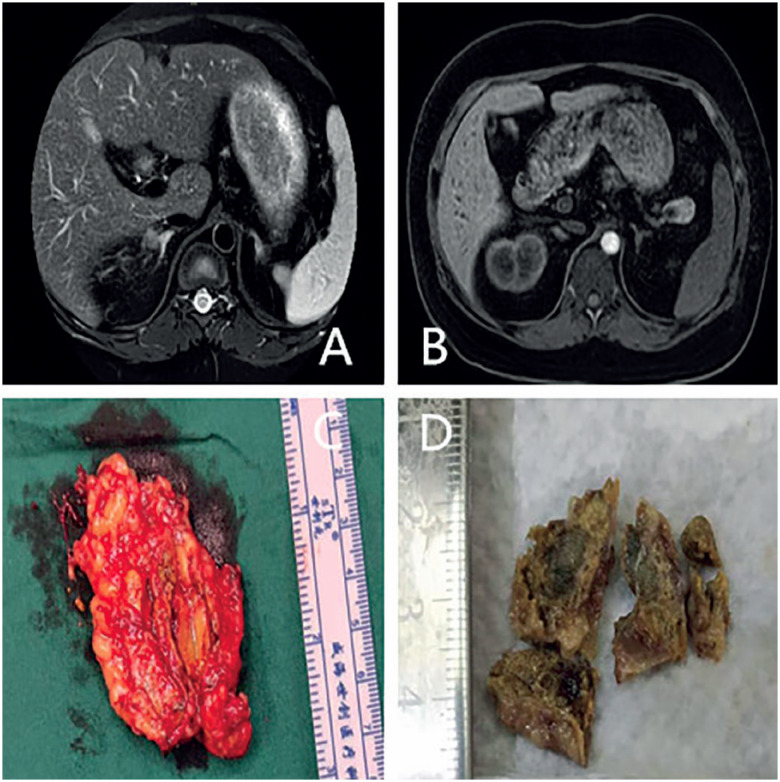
Adrenal MRI findings and gross tissue examination of the resected adrenal gland.
